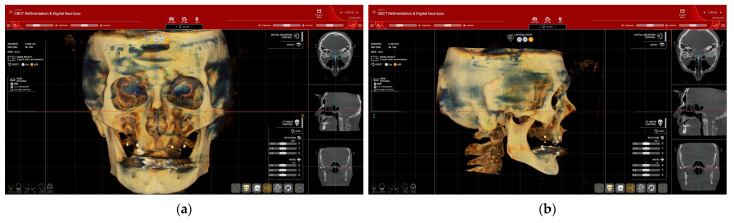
CBCT re-orientation (Patient #1): (a) frontal alignment of CBCT—centering and orienting with the orbital line parallel to the horizontal line; (b) lateral view with the Frankfort plane set as the horizontal plane.
